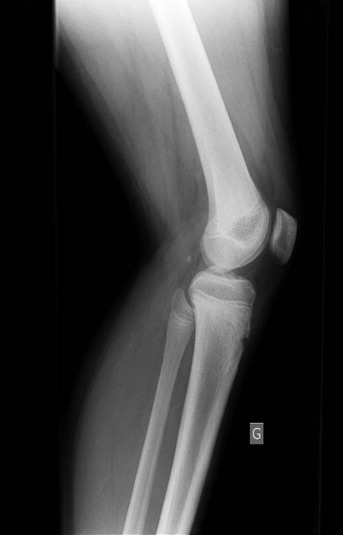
Radiographie de profil du genou gauche
